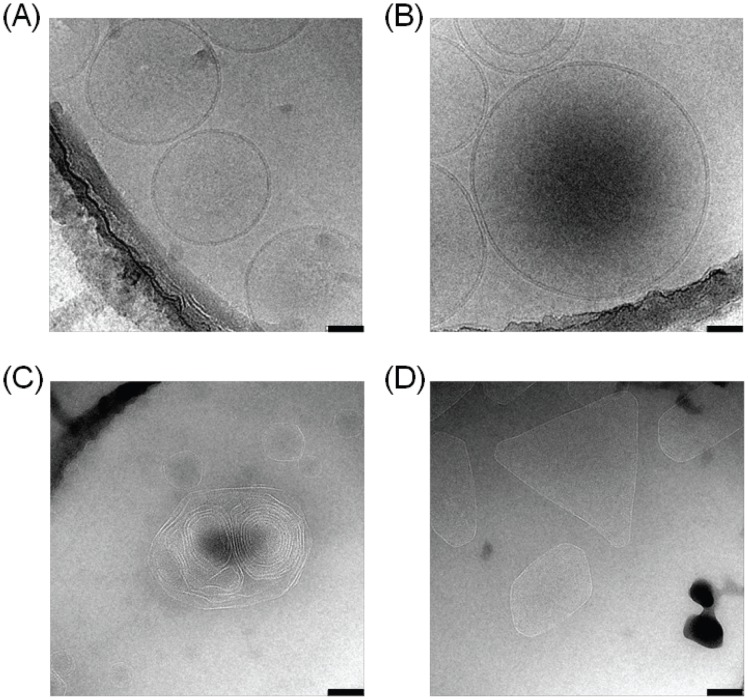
Cryo-TEM images of lipid liposomes prepared in water at 37°C. Liposome of dioleoyl-PC (A and B) or dipalmitoyl-PC (C and D) prepared in water by ultrasonication was observed by cryo-TEM. Scale bars are 50 nm in Figs. A and B and 100 nm in Figs. C and D.
